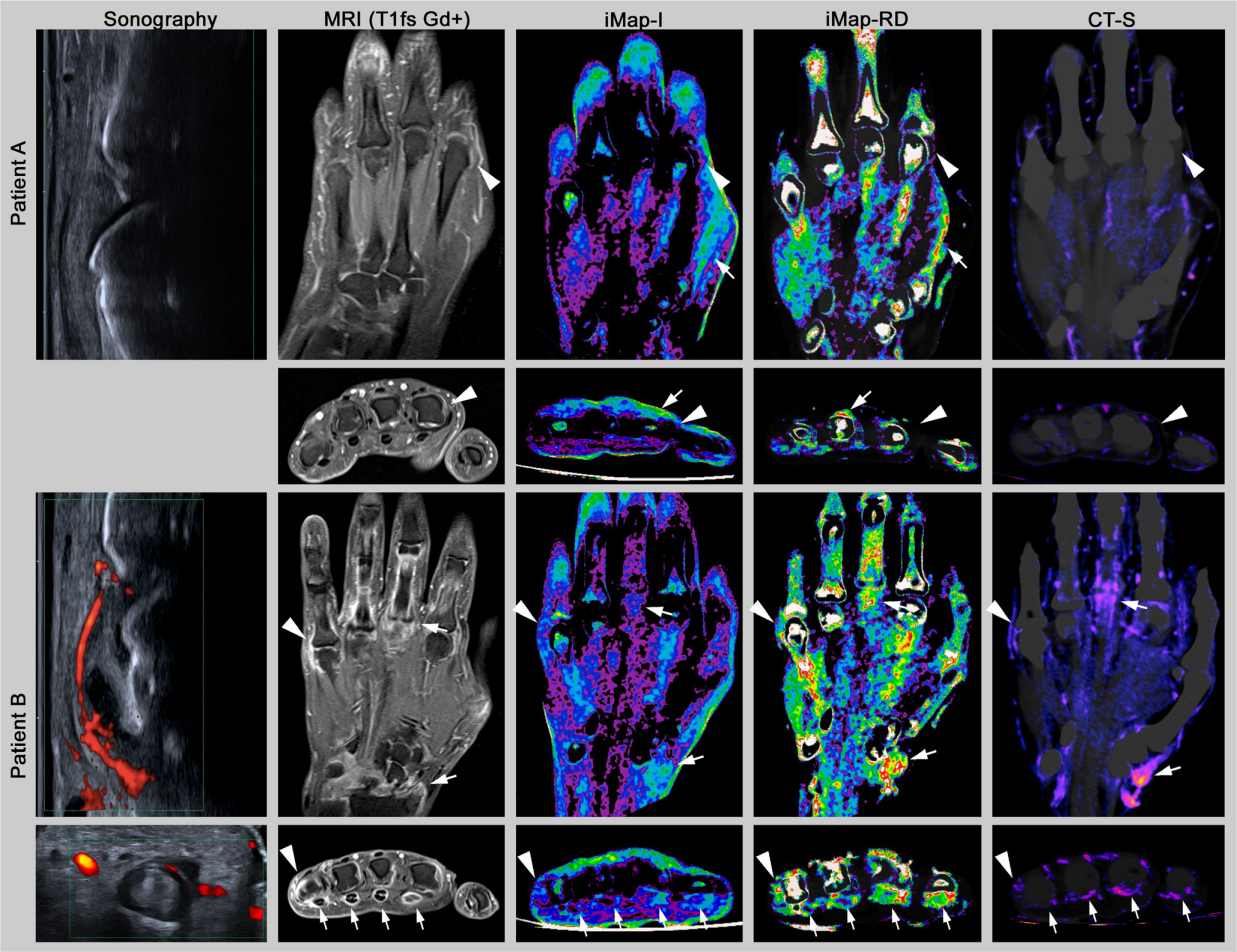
Imaging examples in coronal and axial orientation. MRI (T1fs Gd+) = fat-saturated contrast-enhanced T1-weighted sequence MRI; iMap-I = image data-based dual-energy CT iodine map; iMap-RD = raw data-based dual-energy CT iodine map; CT-S = CT subtraction. Patient A: a 50-year-old female patient with initial seronegative rheumatoid arthritis during therapy. No inflammatory joint changes were detected, e.g., in metacarpophalangeal joint II (MCP) (arrowhead). Note the partial volume effect at the bone and skin border (arrow). Patient B: a 55-year-old female patient with seronegative rheumatoid arthritis. There is severe synovitis in MCP V (arrowhead) and of the radiocarpal joint (arrow). Furthermore, severe tenosynovitis of the flexor tendons II–V was detected in all modalities (arrows), while iMap-I tends to underestimate the tenosynovitis III (arrow) and CT-S tenosynovitis V (arrow)
